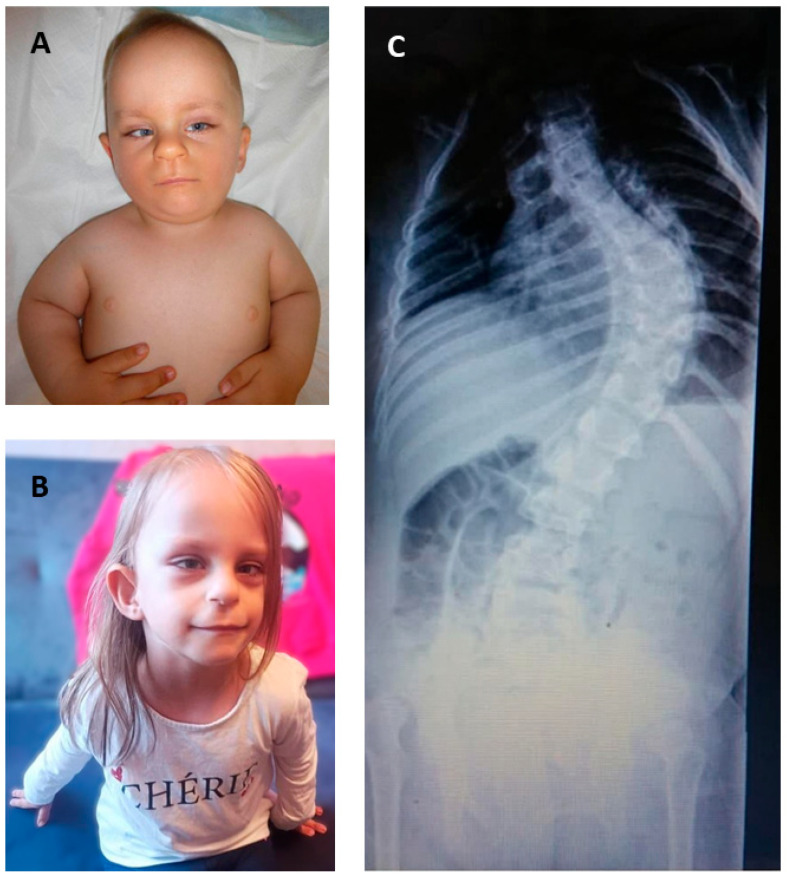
Clinical features observed in the proband. (A) Craniofacial dysmorphism at 20 months of age, including broad nasal bridge, bulbous nasal tip, strabismus, deep philtrum, and low-set ears. (B) Craniofacial appearance at 5years of age. (C) Spinal X-ray showing severe scoliosis.
